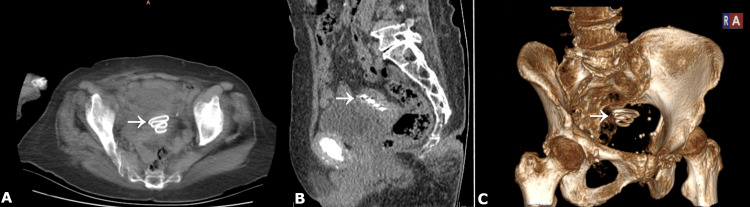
Computed tomography (CT) images of the pelvis demonstrating the intrauterine device (A) Axial minimum-intensity projection, (B) sagittal plane reconstruction, and (C) three-dimensional volume-rendered image confirm the intrauterine location of the device (arrows) within the endometrial cavity, without evidence of extrauterine migration, perforation, or involvement of adjacent pelvic organs.
